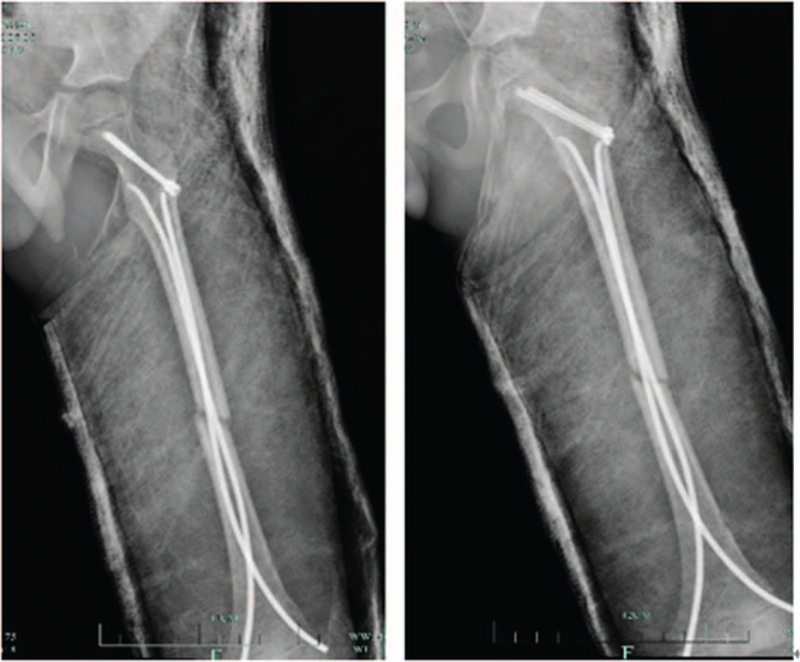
The above picture shows the X-ray findings after surgery.
